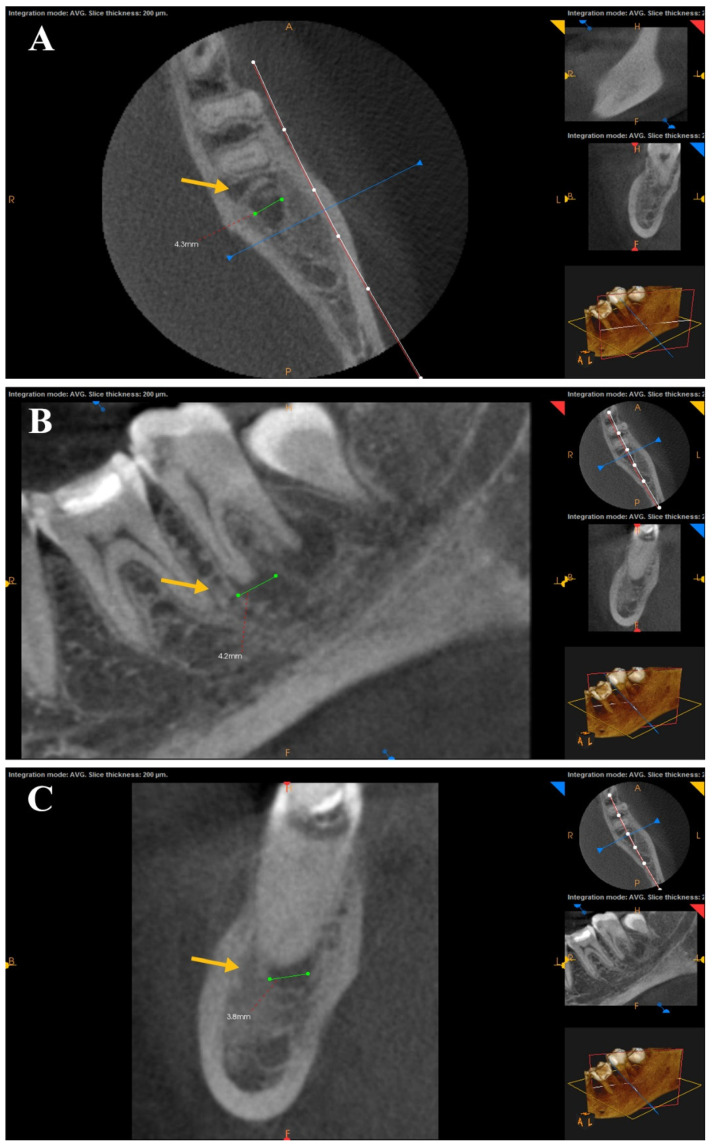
CBCT scans show the axial (A), sagittal (B), and coronal (C) planes. The largest extension of the lesion (yellow arrow) determined the score in the CBCT PAI.
